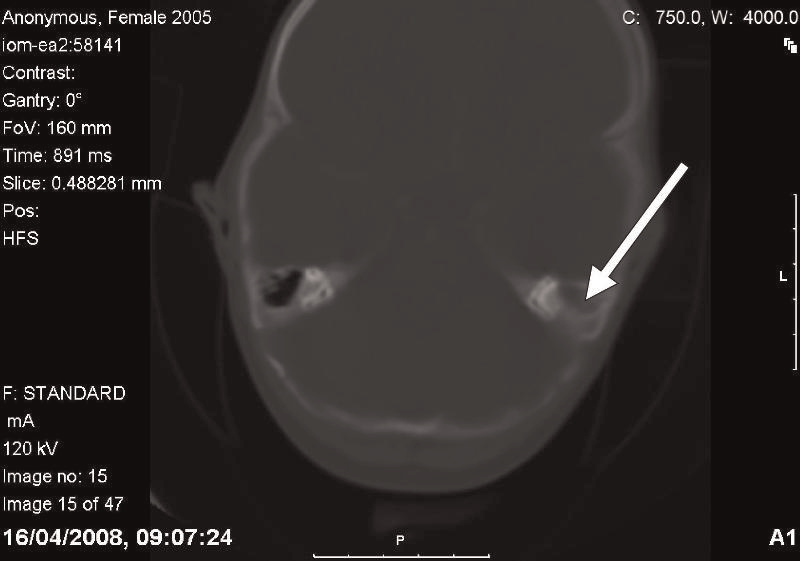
Axial computed tomography scan of the head and neck (taken at time of presentation) through the petrous bones shows osteolysis of the mastoid on the left side, indicating chronic mastoiditis with abscess.
